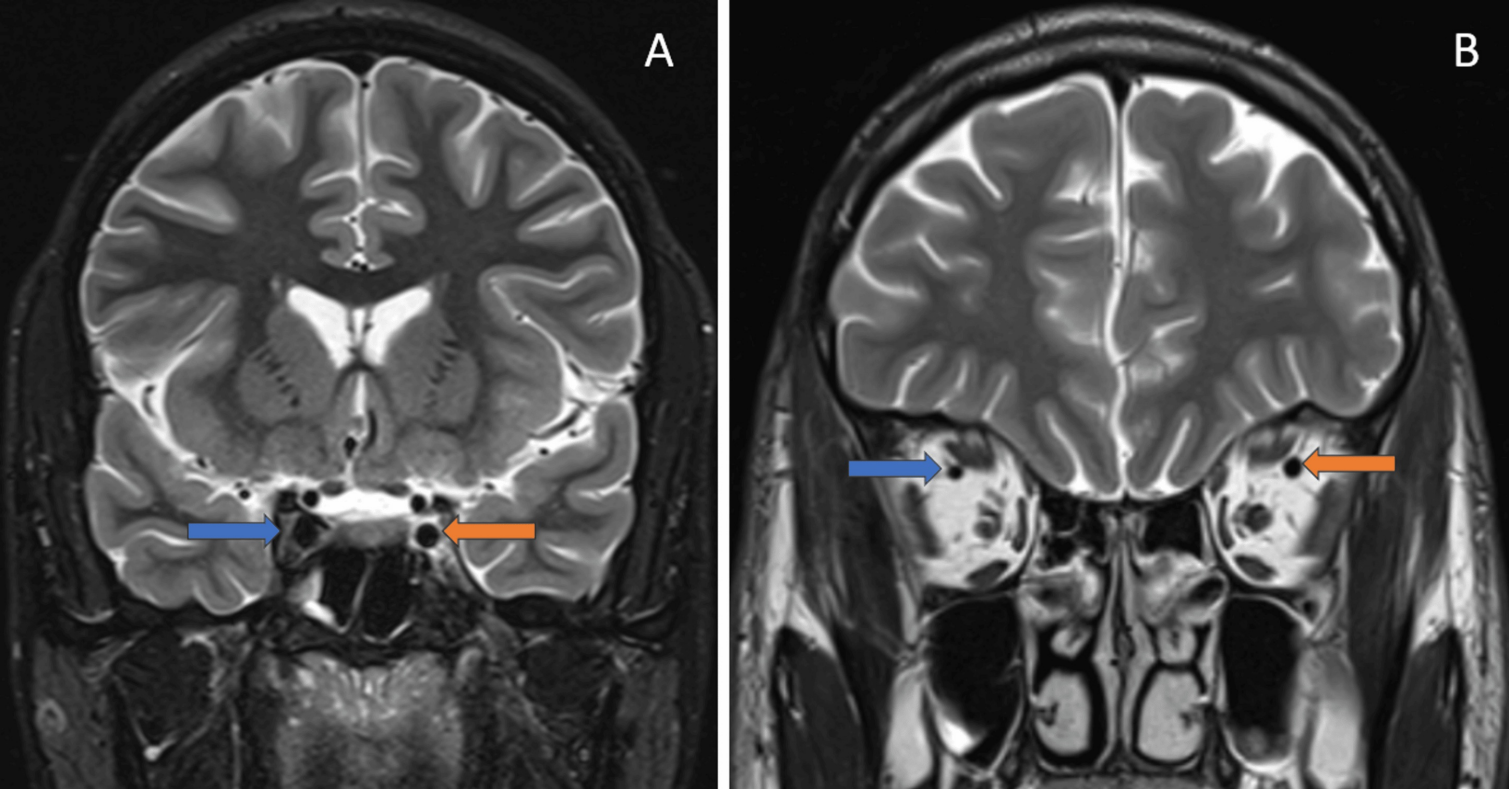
(A) Coronal MRI short tau inversion recovery (STIR) image shows high signal intensity in the left cavernous sinus (orange arrow) compared to the normal signal intensity of the right cavernous sinus (blue arrow). (B) Coronal MRI T2-weighted image shows the prominence of the left superior ophthalmic vein (orange arrow) compared to the normal-sized right superior ophthalmic vein (blue arrow).
